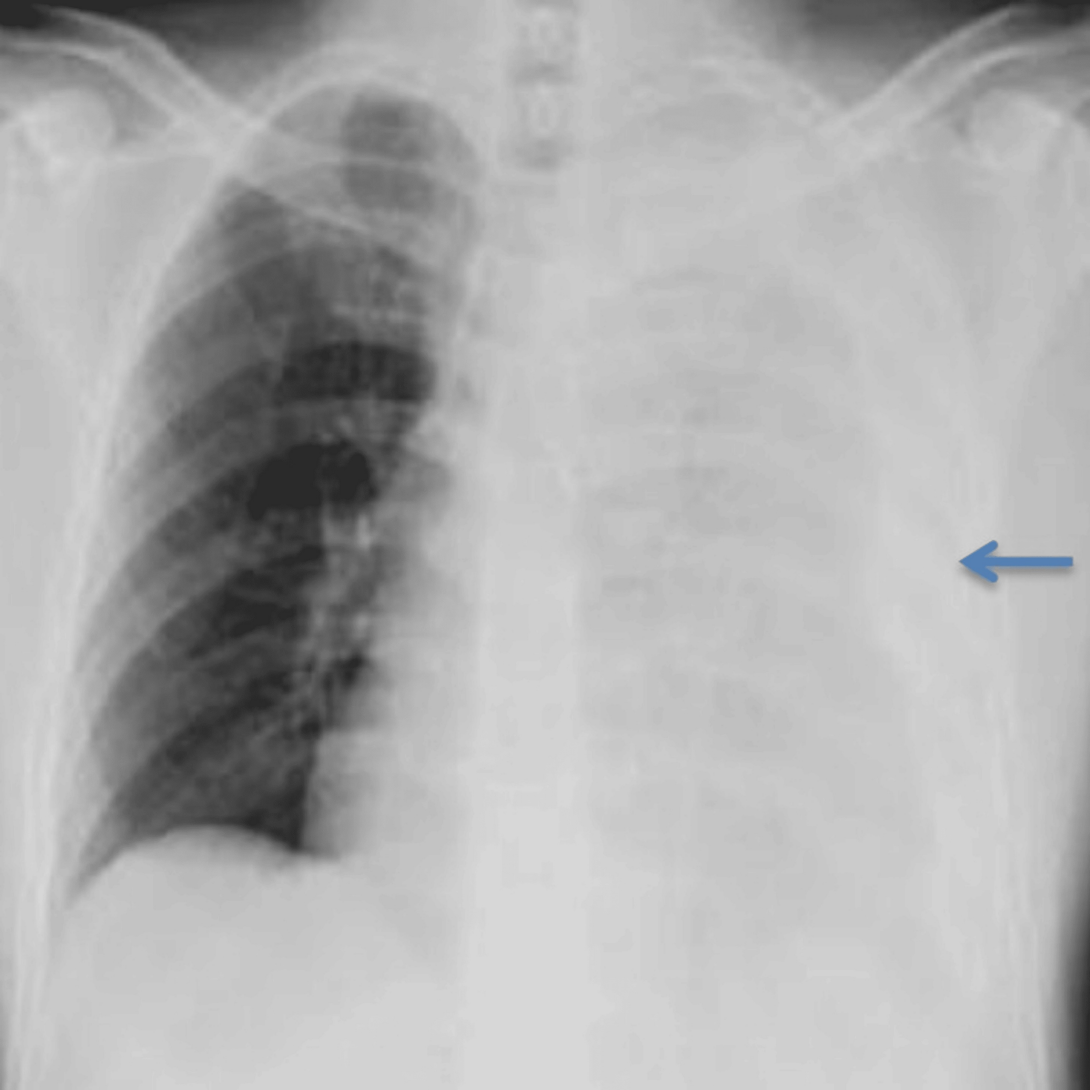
Initial chest X-ray (AP) at presentation showing a left-sided hemothorax with multiple rib fractures (arrow)
